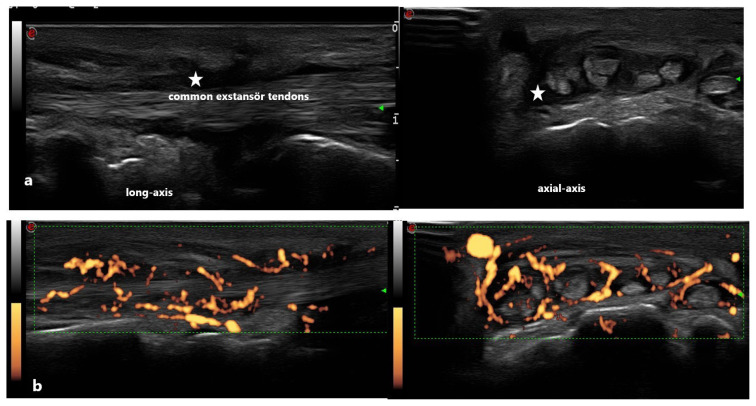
Tenosynovitis image of an RA patient at wrist level shows active (grade 3) tenosynovitis with both axial and longitudinal gray (a) and Doppler scales (b). Stars indicate synovial fluid.
